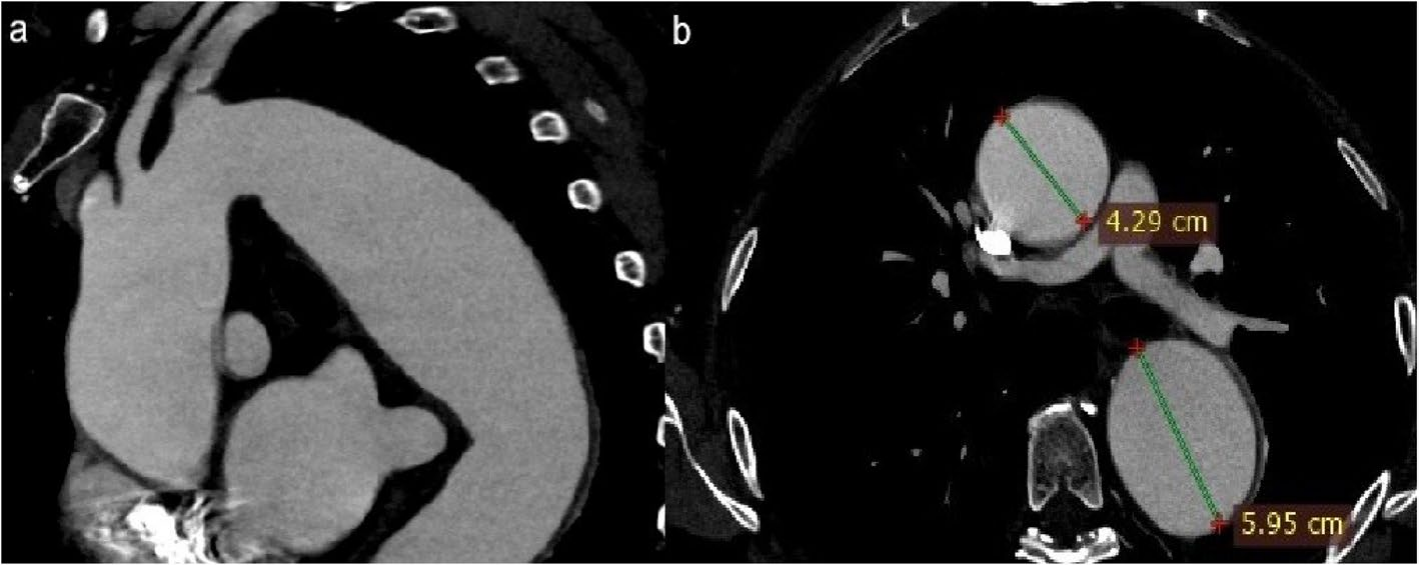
Saggital reconstruction and axial CTA image shows a large descending aorta aneurysm originating very close to the LSA ostium
